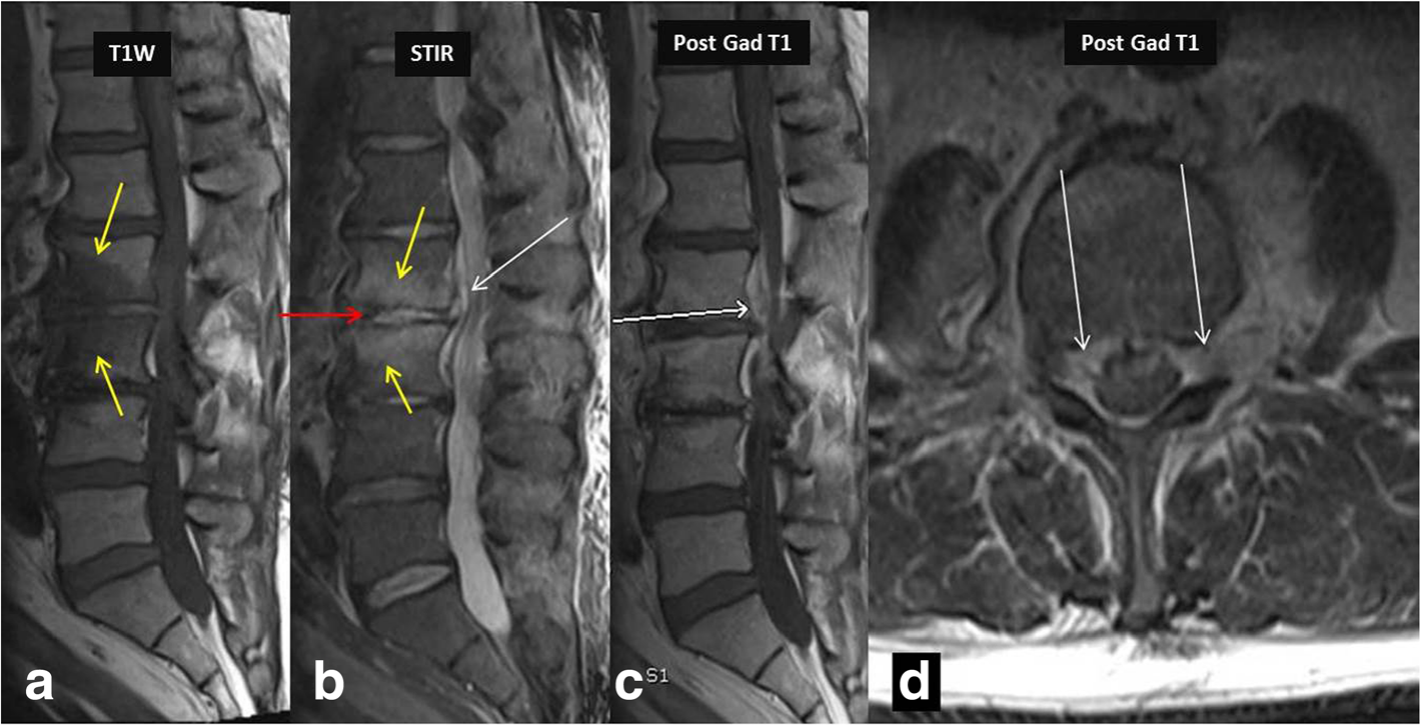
MR imaging in a 36-year-old intravenous drug abuser female shows marrow edema involving L2–3 vertebra reaching up to the endplates (*yellow arrows* in a and b), with minimal enhancement on post contrast imaging consistent with osteomyelitis. Note increased amount of fluid signal in L2–3 intervertebral disc suggesting discitis-osteomyelitis complex. Post contrast imaging shows enhancing epidural soft tissue (*white arrows* in b, c and d) without areas of liquefaction, consistent with epidural phlegmon. Once again post contrast imaging can differentiate epidural abscess from phlegmon and thus alter management. Also note the presence of left psoas muscle involvement. Responsible bacteria in this case were *Staphylococcus epidermidis*
